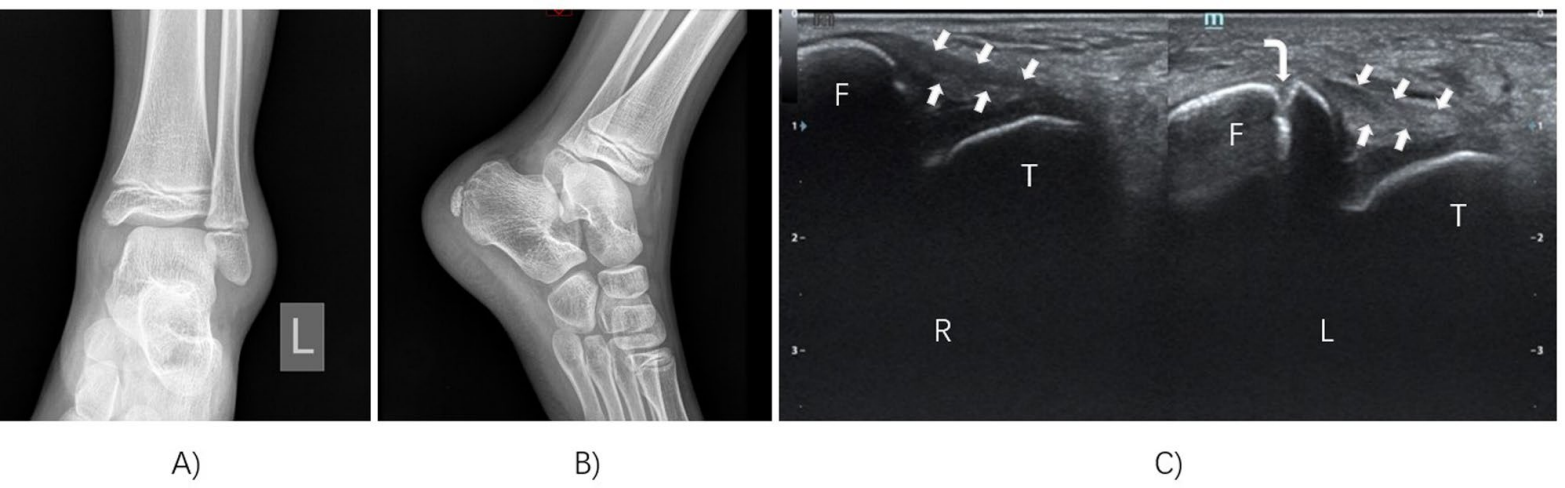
Chondral avulsion fractures of the distal fibula were negative on radiography but positive on ultrasonography. (A) Anteroposterior X-ray radiographs of the ankle. (B) Lateral X-ray radiographs of the ankle. (C) Ultrasound examination of the ankle was performed and compared with the contralateral side. *R*, right; *L*, left; *F*, fibula; *T*, talus; arrowhead, anterior talofibular ligament; right angle arrow, chondral avulsion fracture of the distal fibula
